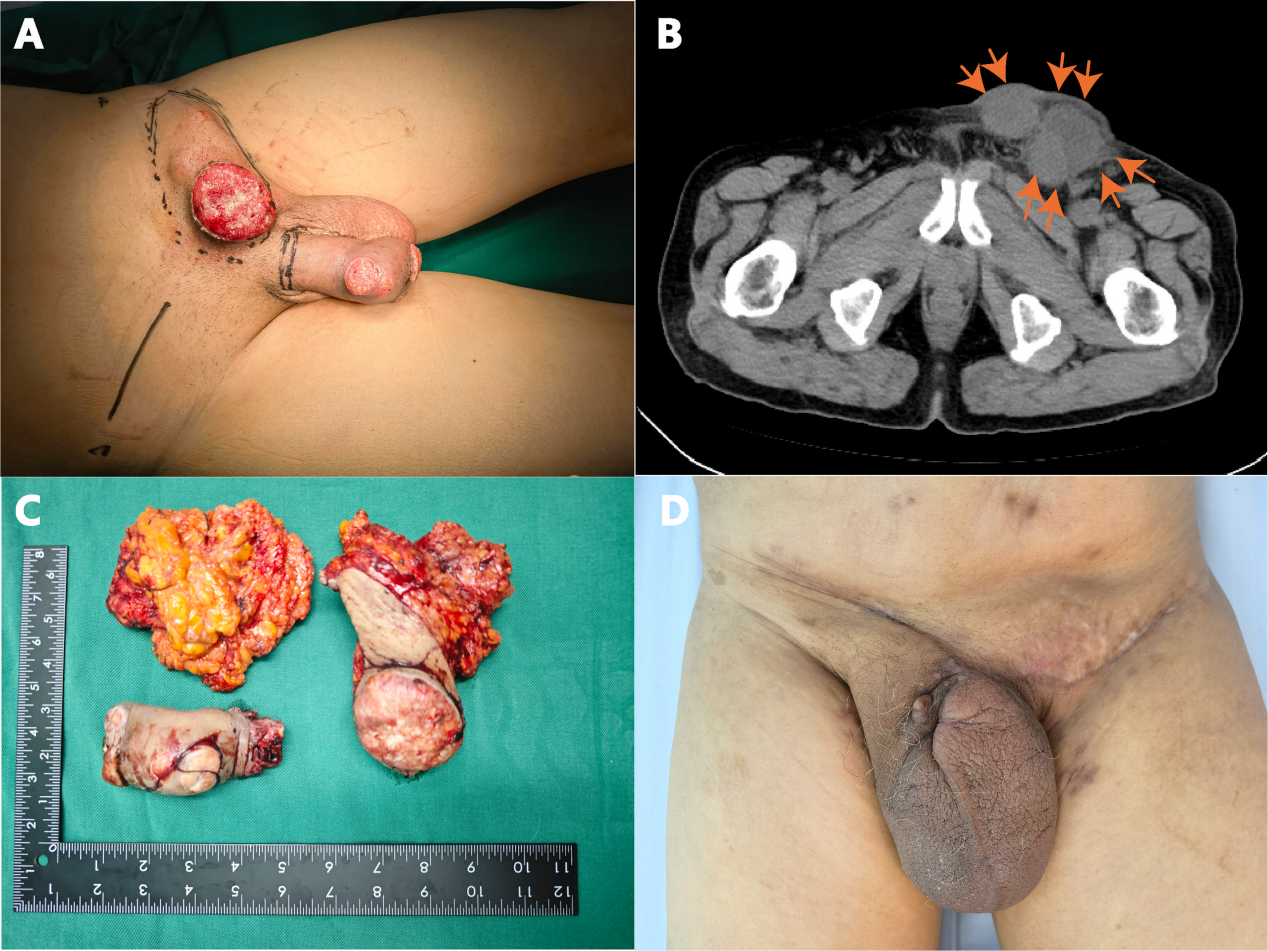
(A) Ulcerative lesion on the glans penis and shaft, accompanied by inguinal lymphadenopathy; (B) CT scan showing enlarged left inguinal lymph nodes; (C) Resected penile tumor and lymph nodes; (D) Surgical site healing at 6 weeks post-operation.
